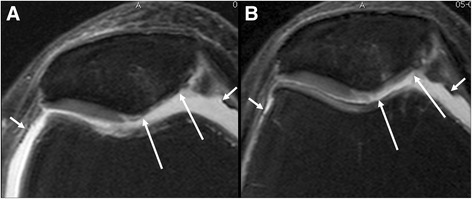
Axial intermediate-weighted fat-suppressed MRI in treated patient at baseline (a) and 12 months (b) follow up show improvement of cartilage focal defect and thickness of the medial patella (long arrows). Also note the decrease in volume of the joint effusion (small arrows)
